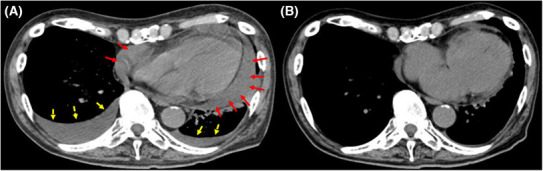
(A) Transaxial plain CT at the initial visit showed diffuse pericardial effusion (red arrows) and pleural effusion (yellow interrupted arrows). (B) CT after pericardial drainage. Pericardial effusion is clearly reduced.
